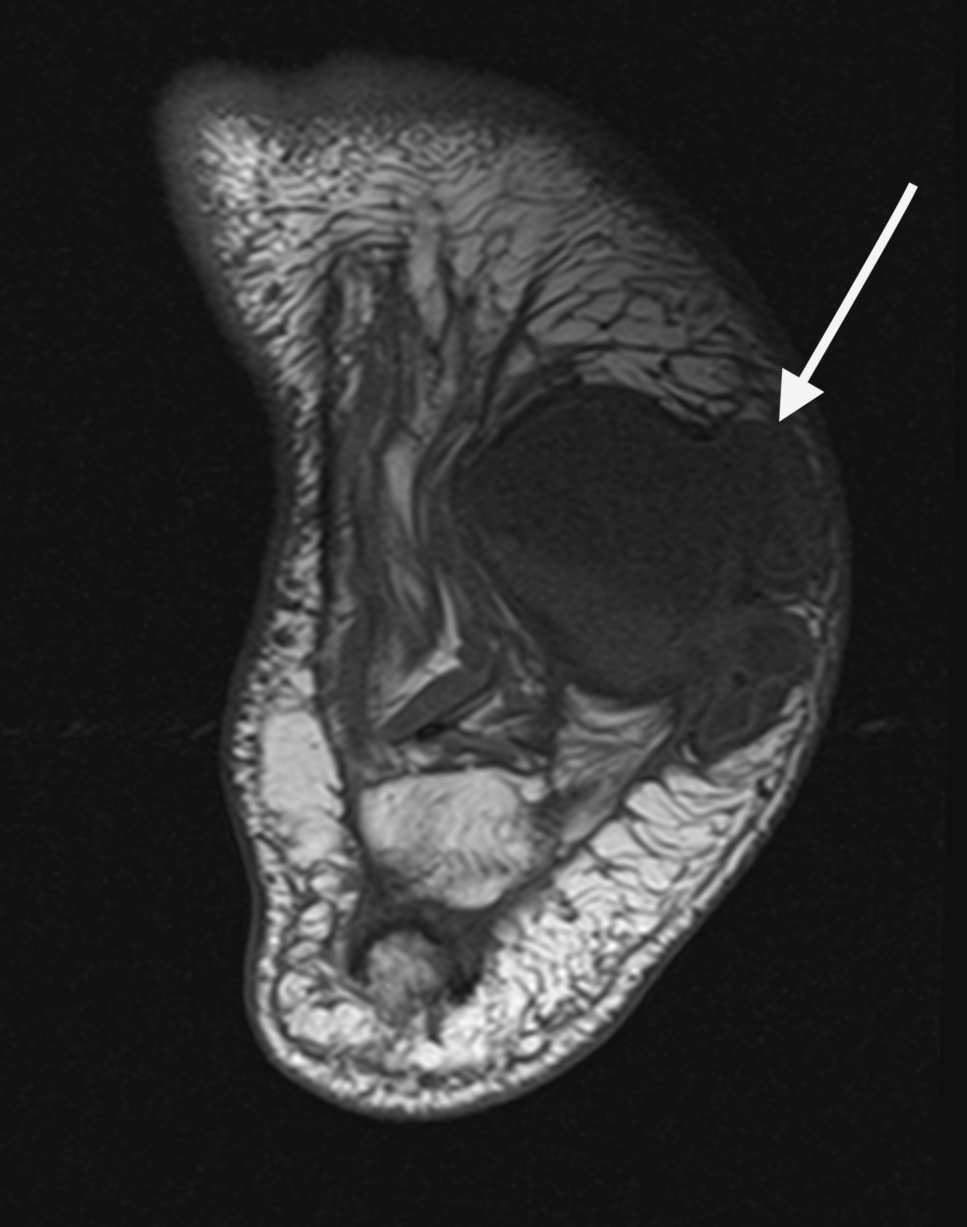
Axial MRI revealed well-organized fluid filled lesion within the plantar lateral tissue that is communicating with the disrupted joints of the midfoot.
